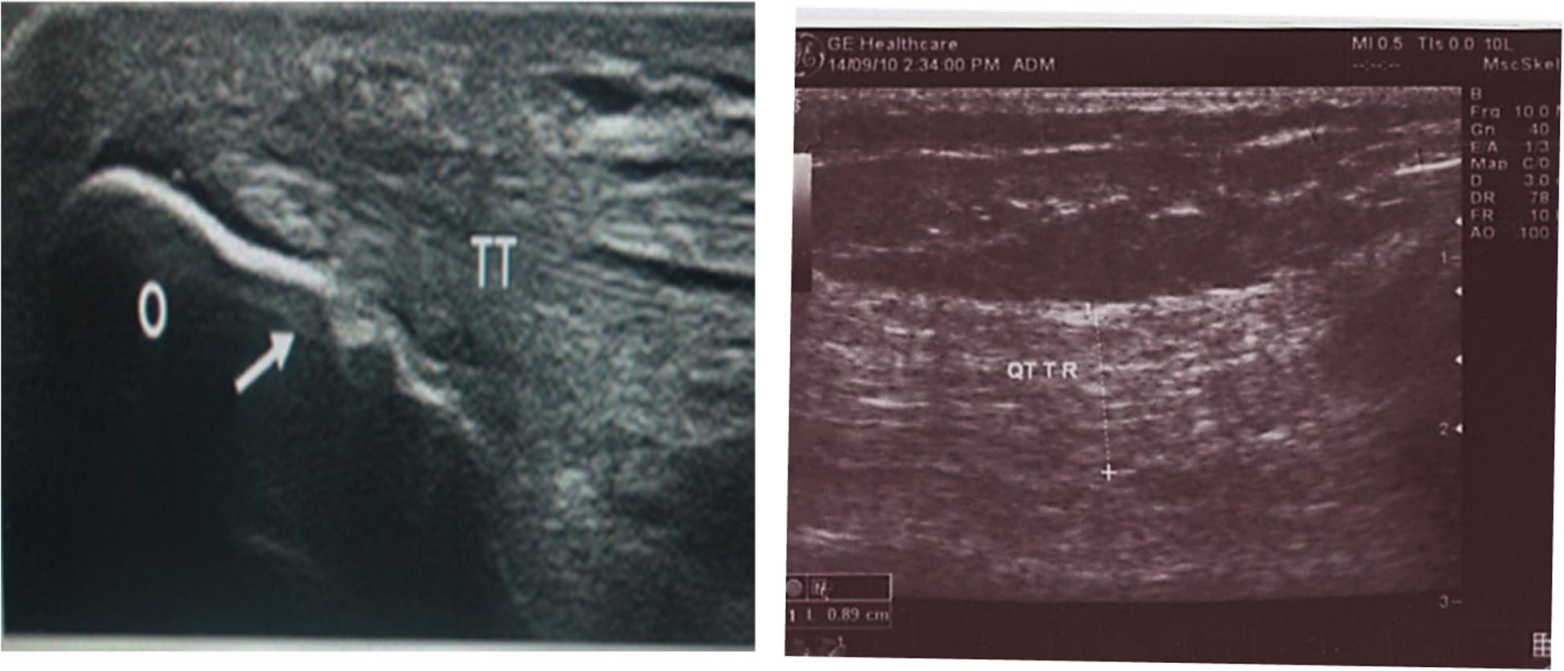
A Longitudinal B-mode superficial ultrasound of the elbow at the site of insertion of triceps tendon (TT) at the olecranon (O) showing irregularities and erosion of the olecranon (white arrow). B Longitudinal B-mode superficial ultrasound at the level of the knee joint showing thickened right quadriceps tendon (QT) (8.9 mm)
